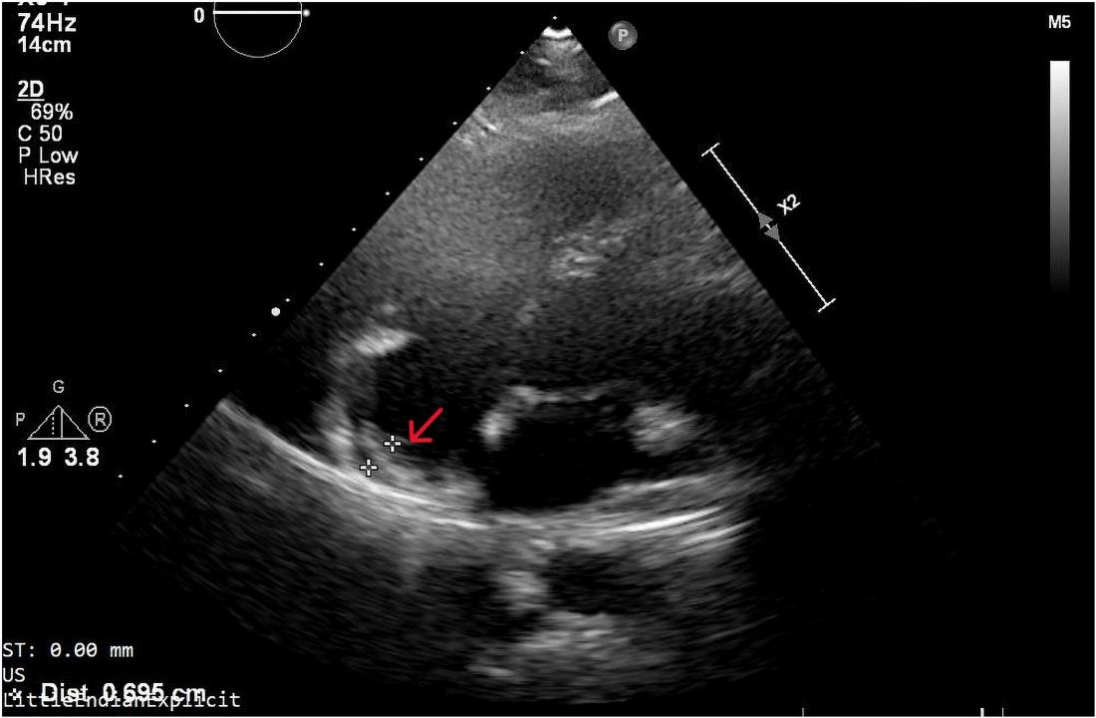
Transthoracic echocardiography; right atrium with hyperechogenic walls, and a mass of ∼7 mm on the superior wall extending to the interatrial septum, indicated by the red arrow.
